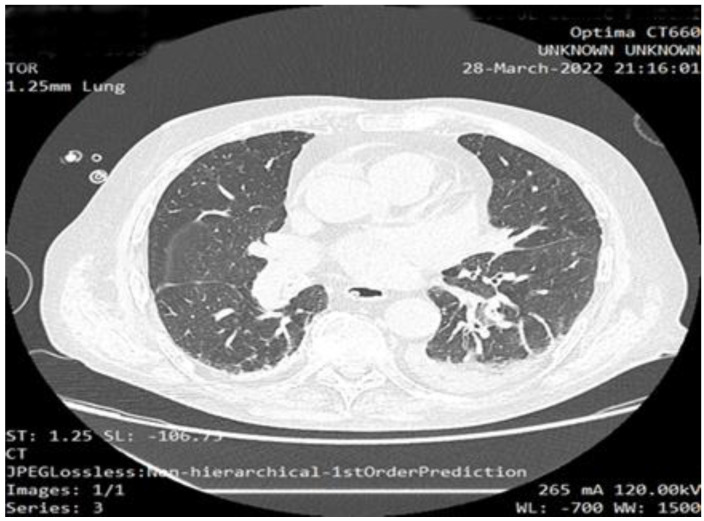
Native CT-scan revealing mild form of COVID-19 pneumonia.
